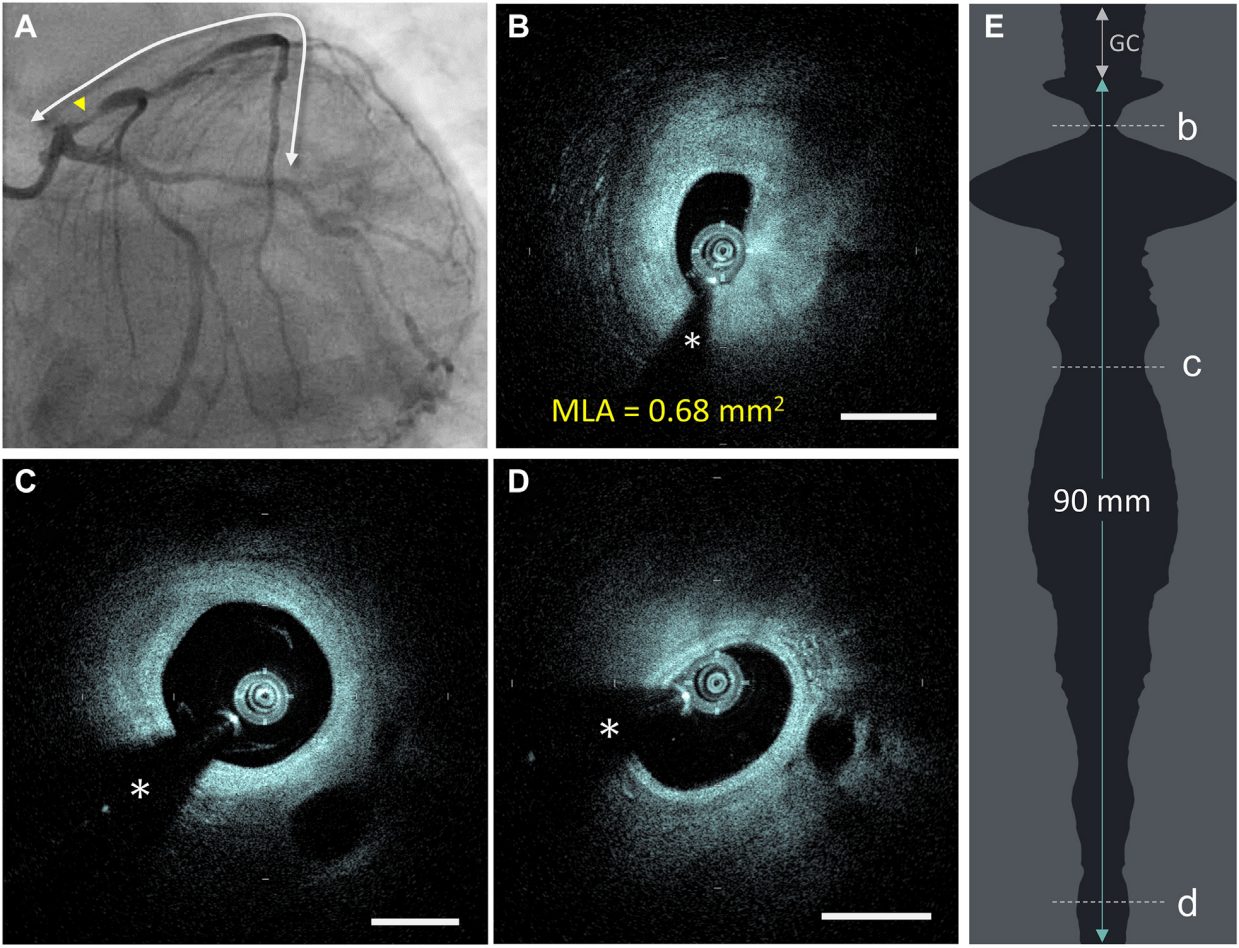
The images show an example of high-frequency optical coherence tomography (HF-OCT) preintervention imaging in a severely stenosed coronary artery. (A) X-ray angiography shows a 90% lesion at the level of the proximal left anterior descending artery. (B) The HF-OCT cross-sectional image shows the MLA site, with a lumen size of 0.68 mm^2^. (C, D) the images show complete blood clearance proximal and distal to the MLA location, offering a complete assessment of the artery disease. (E) The image shows the HF-OCT lumen profile. A 90 mm long clear segment of the artery was obtained in 1 second by injecting contrast using a 10-cc manual syringe injection. Scale bars on all images are equal to 1 mm. The asterisk (∗) indicates the guide wire shadowing artifact. GC, guide catheter; MLA, minimum lumen area.
